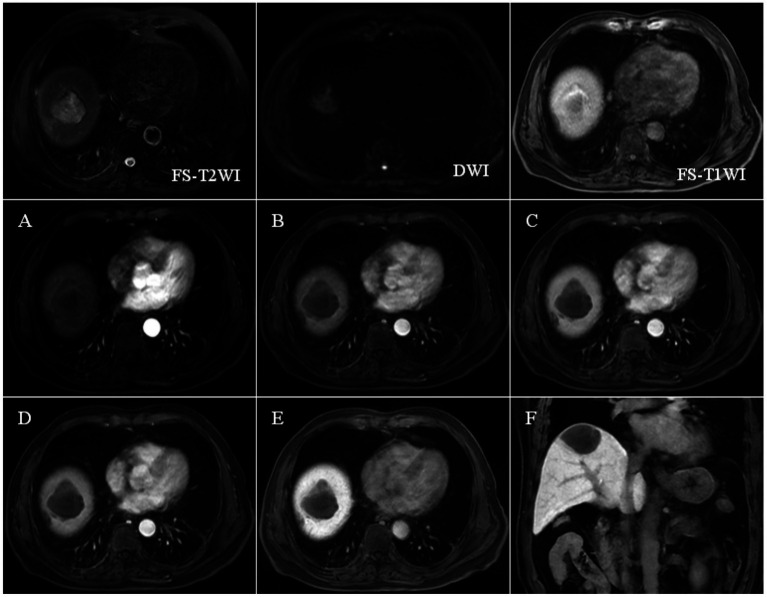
FS-T2WI and DWI: patchy high signal at the top of the right lobe of the liver: FS-T1WI: patchy high signal is seen at the top of the right lobe of the liver, and a patchy slightly low signal is seen within it. Panels (A–F) show the early arterial, late arterial, portal venous, delayed, hepatobiliary axial and coronal positions. EOB-MRI enhancement scans can accurately show the enhancement of the lesion after TACE: the necrotic area shows high signal in T1WI, low signal in T2WI and DWI, and the enhancement scans do not show any enhancement, and the hepatobiliary phase shows a low signal, which is a manifestation of coagulative necrosis.
